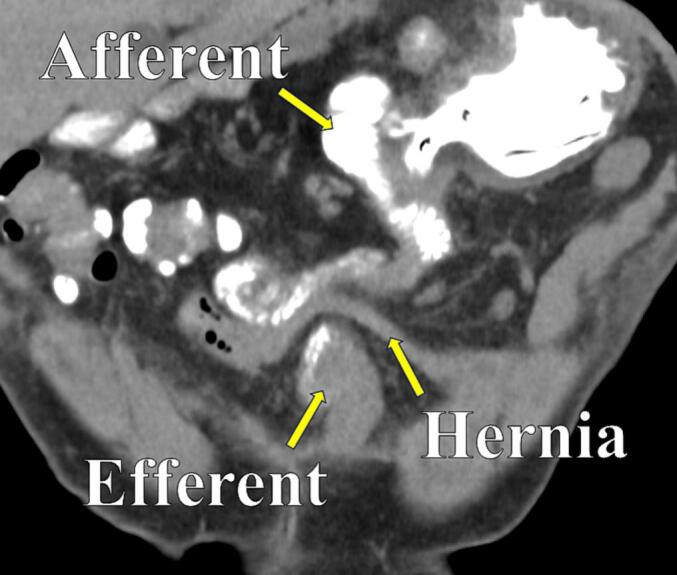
Abdominal computed tomography image revealing an internal hernia.
